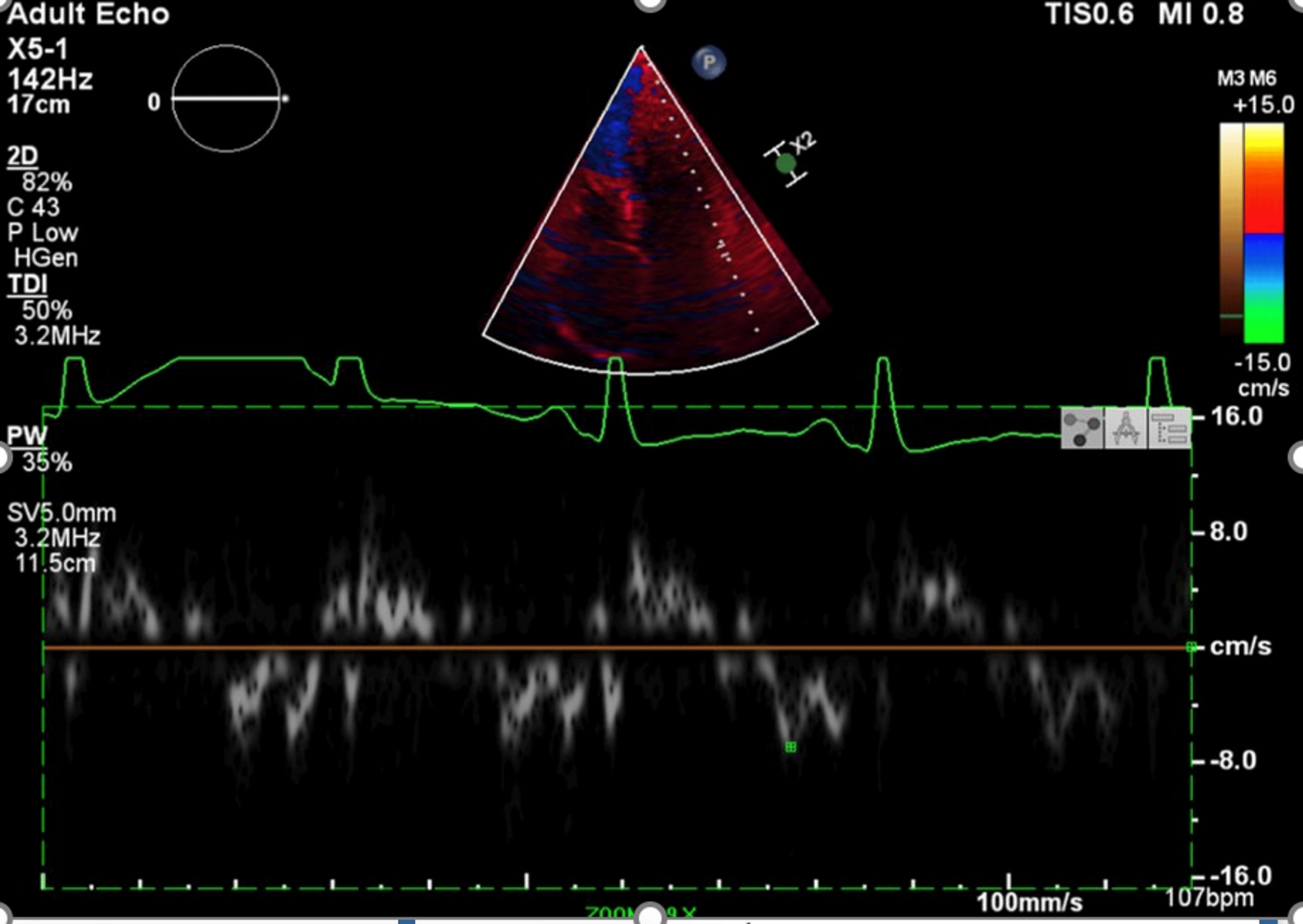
Tissue Doppler imaging from the apical four-chamber view at the lateral mitral annulus The early diastolic velocity (e') measures approximately 8 cm/s. The septal e' velocity (10 cm/s) exceeding the lateral e' velocity (8 cm/s) demonstrates annulus reversus, consistent with constrictive pericarditis
